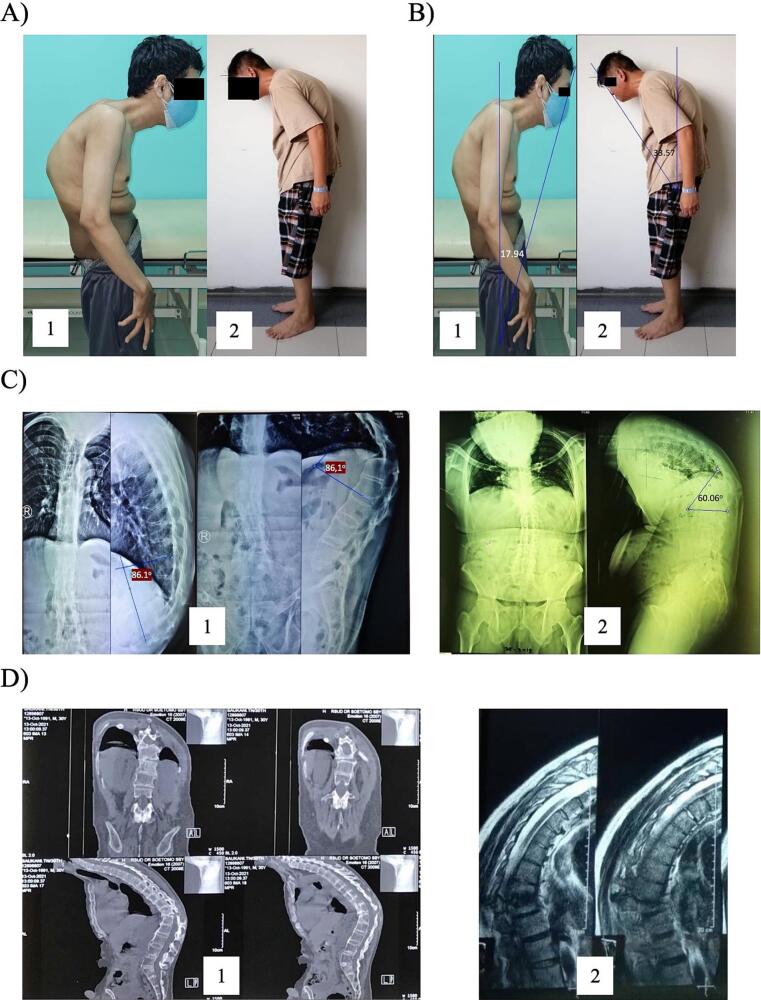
The initial assessment of the patients: (A) the clinical picture, (B) CBVA angle measurements, (C) radiographic plain X-ray in AP and lateral views, (D) CT-scan and MRI evaluations. Note: 1: case 1; 2: case 2.
